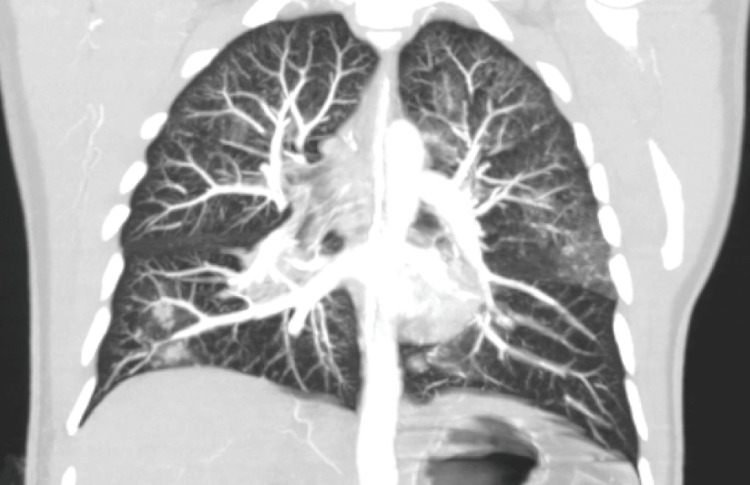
CT pulmonary angiography (coronal MIP, 10-mm slab) on ICU day 4 Very distal SSPE (arrow) seen along a peripheral branch; central and lobar arteries are patent. Coronal MIP enhances visualization of small, contrast-opacified vessels. MIP: maximum intensity projection; SSPE: subsegmental pulmonary embolism
